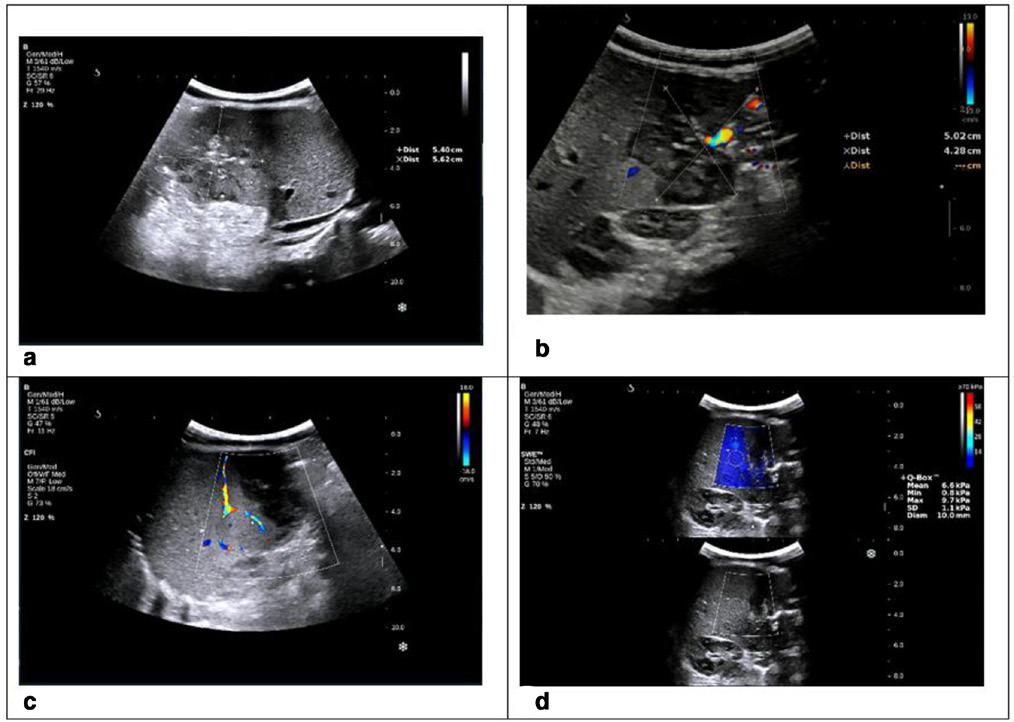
Abdominal ultrasound revealed a right hepatic lesion with mixed echo, multi curvilinear, (a, b) Mode ultrasound section showed right hepatic lesion, segment VI with many echogenic septae inside, b&c: Doppler ultrasound showed solid content with vessels, d: Real-time 2D shearwave elastography (SSI) showed solid content with low stiffness around 6.6 kPa.
